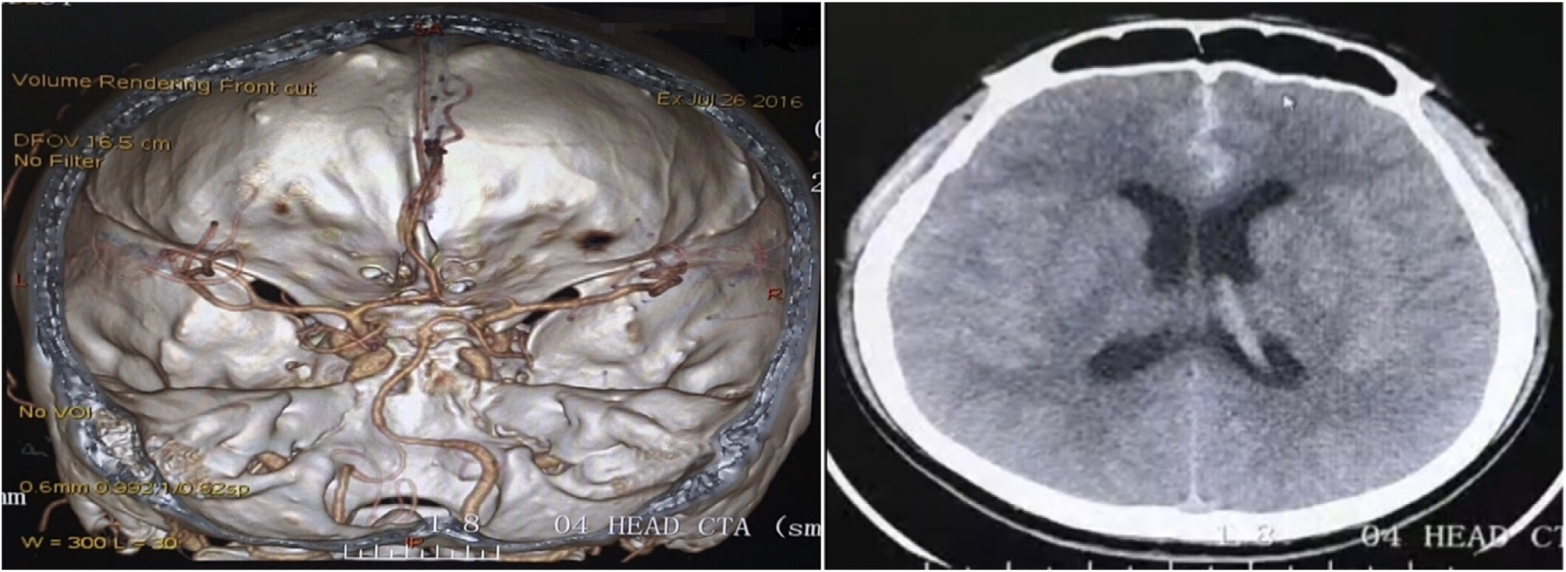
Computed tomography angiography revealed an anterior communicating aneurysm with a subarachnoid hemorrhage
